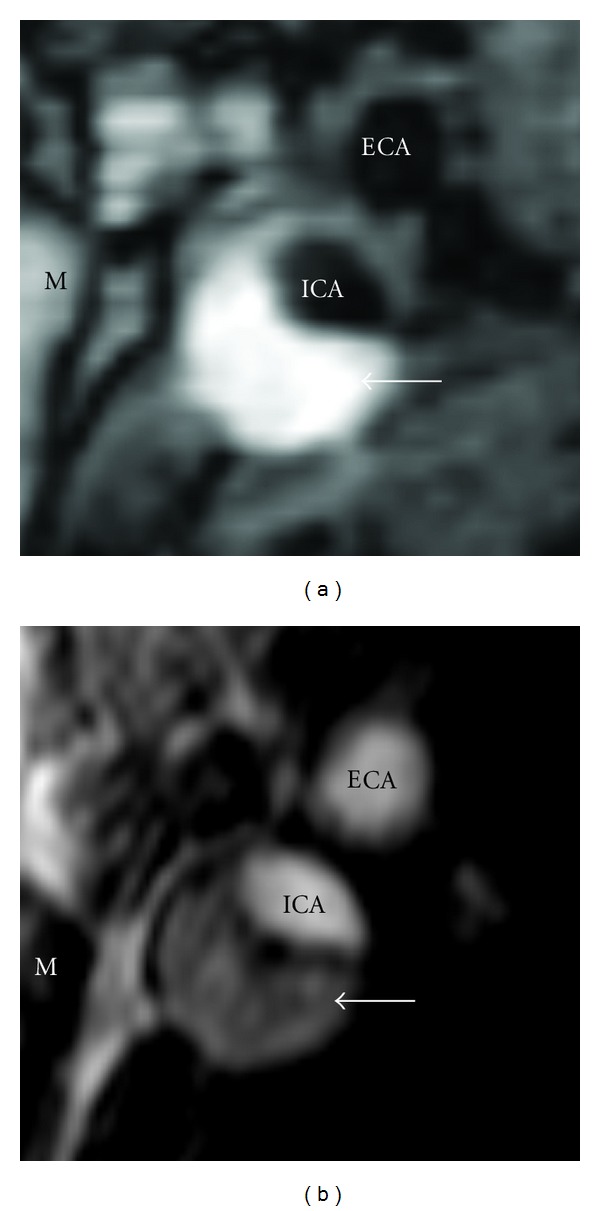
Appearance of intraplaque hemorrhage (arrow) on (a) T1-weighted fast spin-echo and (b) time-of-flight magnetic resonance images.
